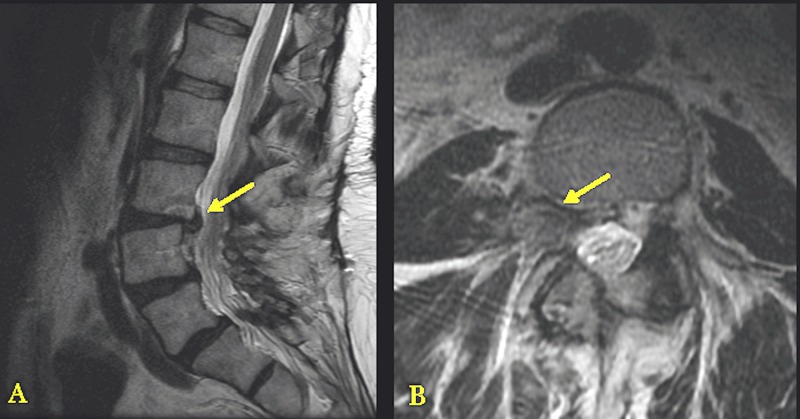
Preoperative Magnetic Resonance Imaging (MRI) Panel A demonstrates lateral MRI with the arrow pointing to the disc herniation at L3-L4. Panel B demonstrates an axial MRI with the arrow pointing to the disc herniation with nerve root impingement at L3-L4.
